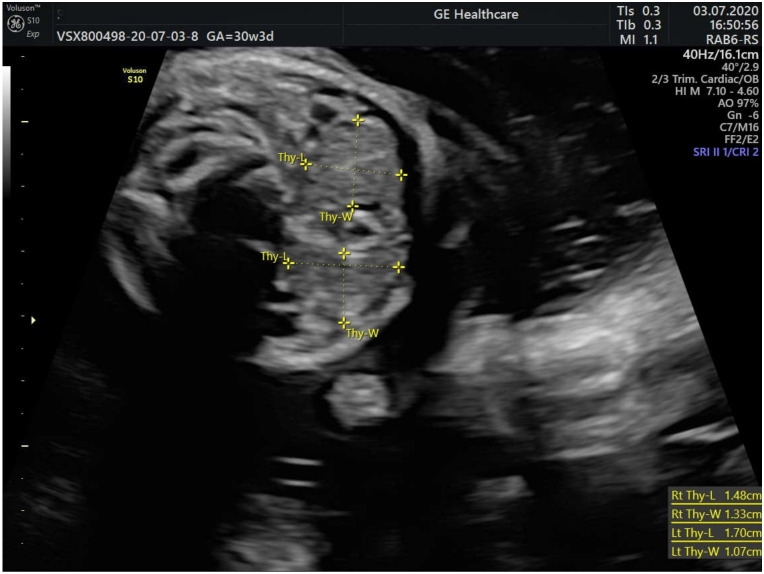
2-D Ultrasonographic measurements of the fetal thyroid at 30 weeks and 3 days of gestation: right thyroid lobe measures 1.48/1.33 cm and the left thyroid lobe measures 1.70/1.07 cm.
